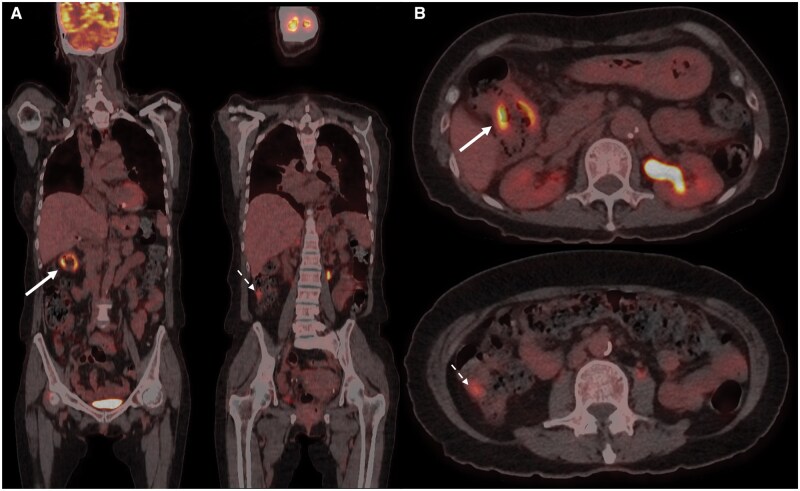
Repeat [18F]-FDG PET/CT performed 6 months later with (A) coronal fused PET/CT, (B) and axial fused PET/CT showed persistent, intense uptake at the hepatic flexure (solid arrow) and a new, moderately avid focus more inferiorly (broken arrow). The uptake within the liver and lymph nodes was no longer visualized (not shown).
